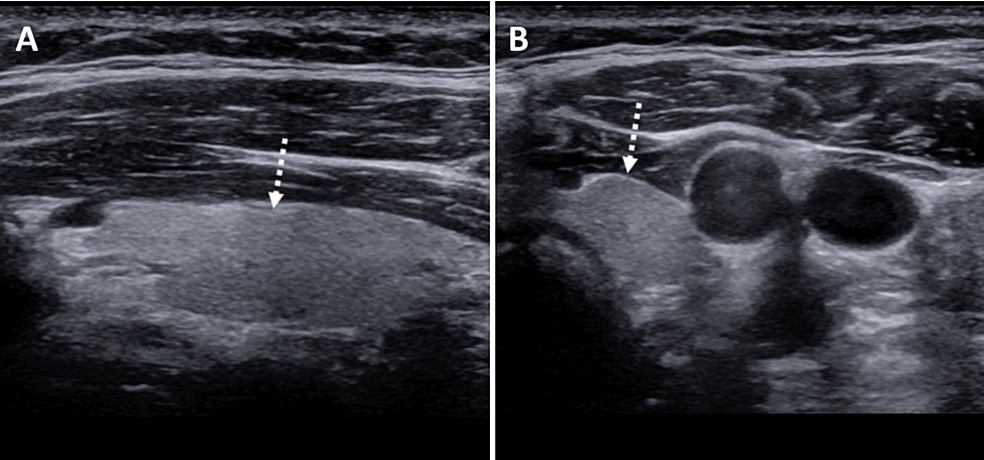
Ultrasound of the soft tissue of the neck (A) longitudinal and (B) transverse views of the left thyroid lobe shows an unremarkable left thyroid lobe (dashed white arrows) with no ultrasound evidence of a parathyroid nodule.
